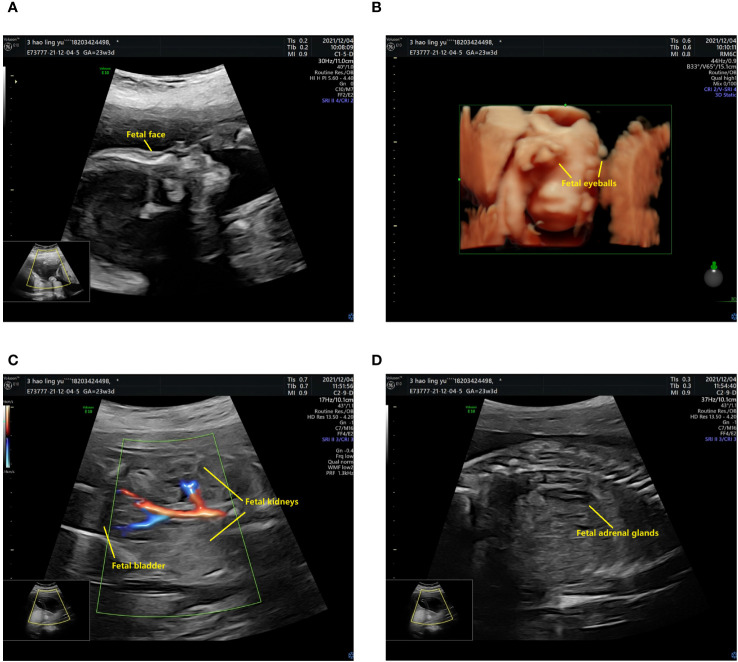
The prenatal ultrasound findings of the patient. (A) The fetal head and face exhibit several notable features. The skull is clearly discernible, with the midline of the brain appropriately aligned. The thalamus is visible on both sides, and the morphology of the cerebellar hemispheres appears normal without any evident abnormalities. Additionally, the cerebellar earthworms are visible, and there is no significant enlargement observed in the pool of the posterior cranial fossa. (B) The presence of bilateral eyeballs in the fetus is evident, and there is no apparent disruption in the continuity of the skin echoes of the upper lip. (C) The fetal abdomen and viscera exhibit visibility of the liver, stomach, left kidney, and bladder, with no apparent disruption in the echogenicity of the abdominal wall. The left kidney measures 33x14 mm in size, with a separated left renal pelvis measuring approximately 5 mm in width. There is no notable renal echo in the right renal region or pelvic cavity, and the right adrenal gland displays a “lying down” sign. (D) CDFI imaging reveals only the left kidney, while the fetal cord consists of two umbilical arteries.
